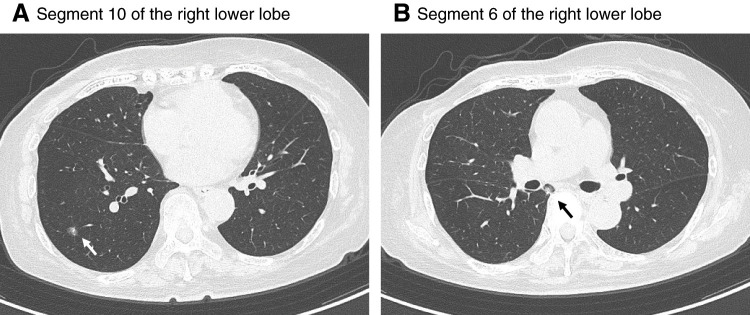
Preoperative chest CT images. (A) A slowly enlarging part-solid ground-glass opacity was identified in segment 10 of the right lower lobe, measuring 7.3 mm in total diameter with a 1-mm solid component. (B) A newly appeared solid nodule measuring approximately 10 mm was observed in segment 6 of the right lower lobe (arrow).
